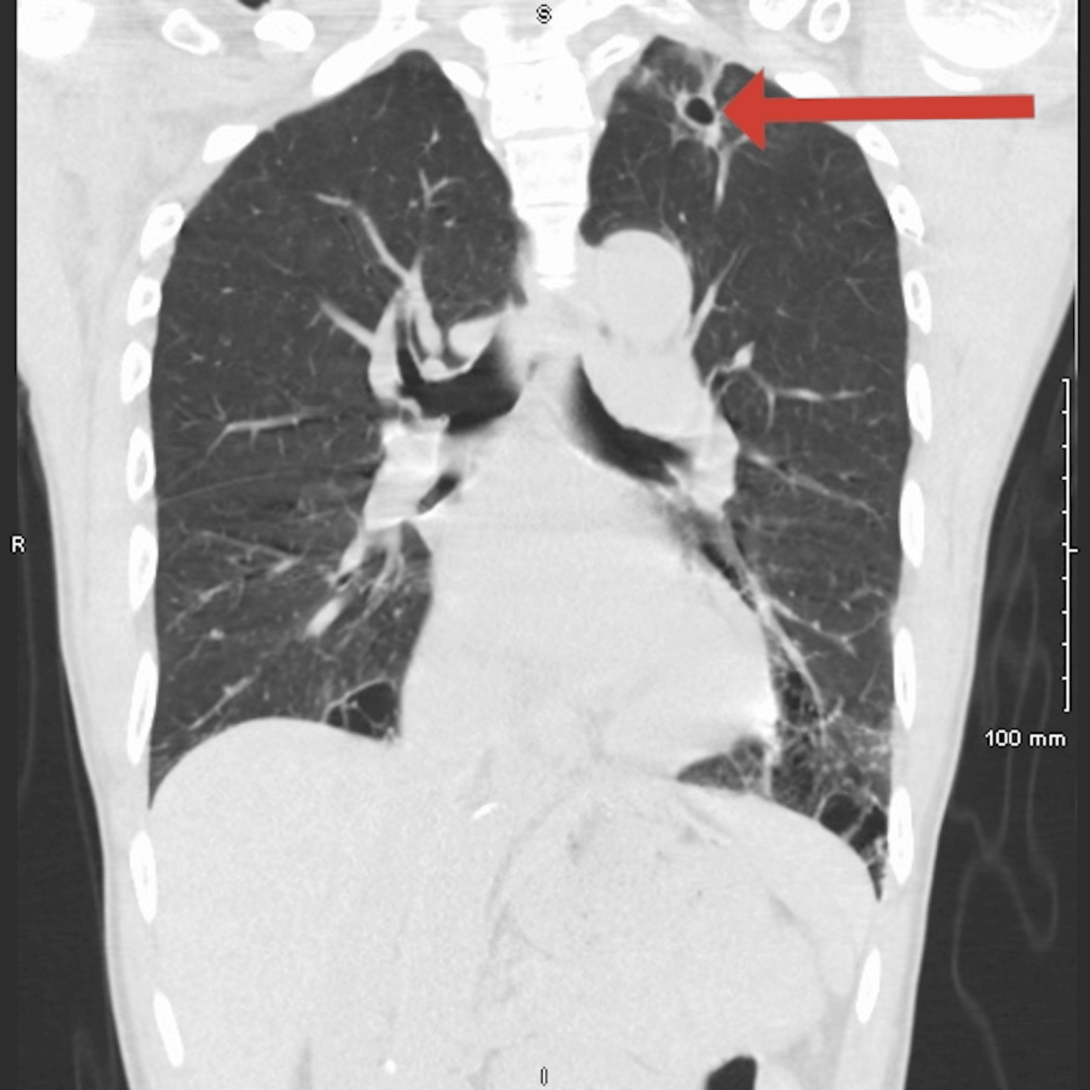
Non-contrast CT image of the chest demonstrating a slightly decreased left apical cavitary lesion, as indicated by the red arrow
